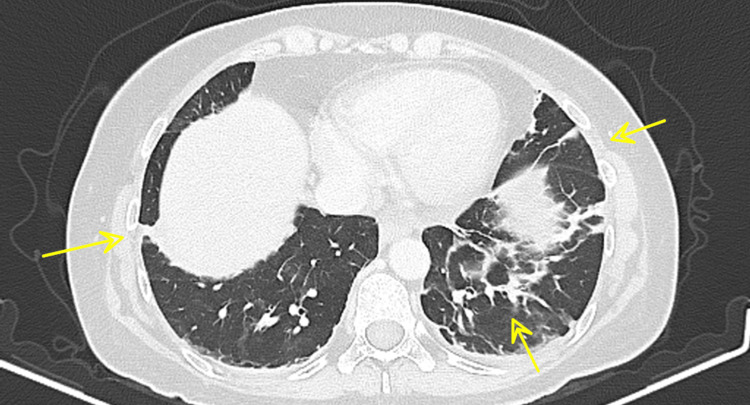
Chest CT 10 days after pembrolizumab administration Additional band-like atelectatic changes involving the lingula and both lower lobes (yellow arrows). CT: computed tomography
